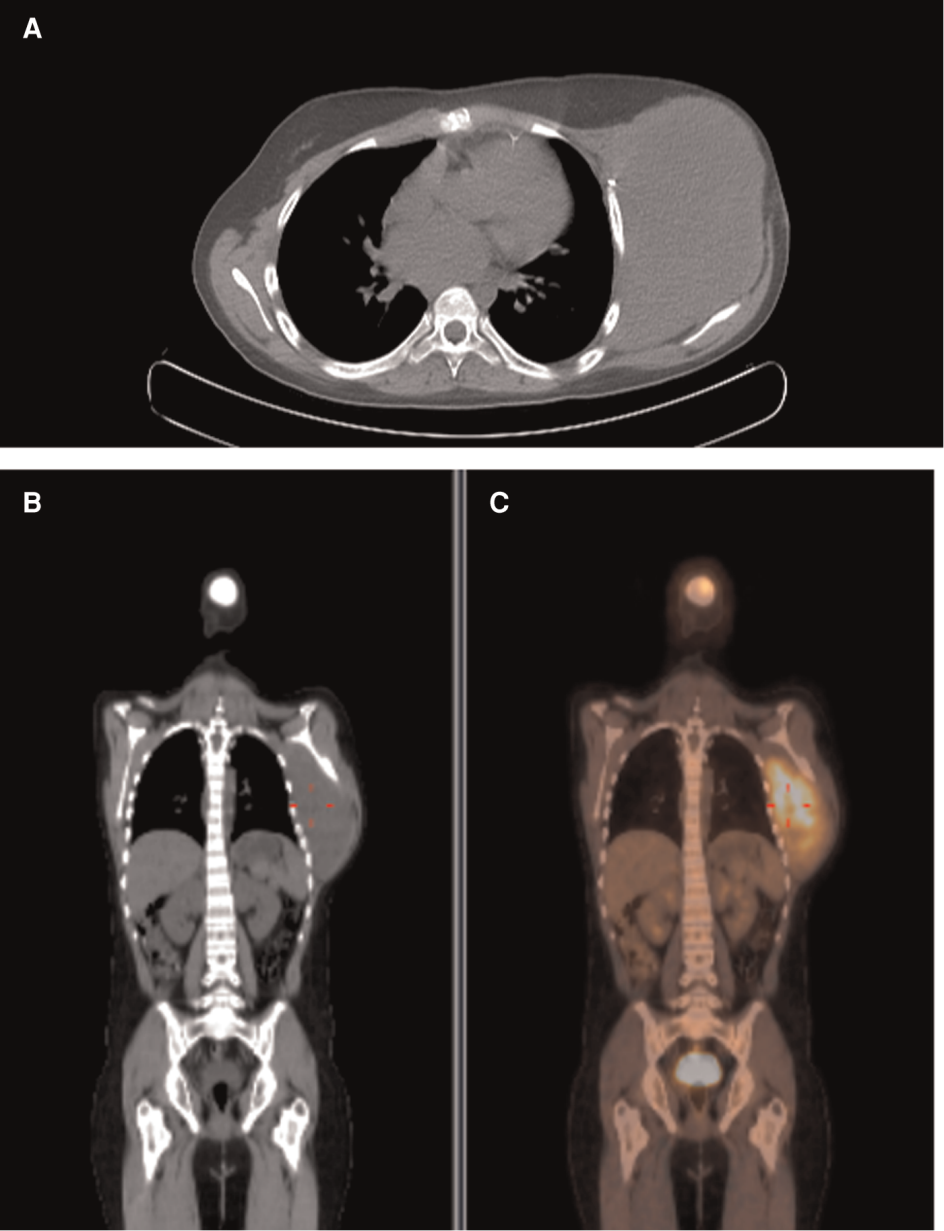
Preoperative PET image. Captant area in thoracic wall with SUVmax: 7.8. (A) Axial image. (B) Coronal image. (C) Coronal fusion image.
